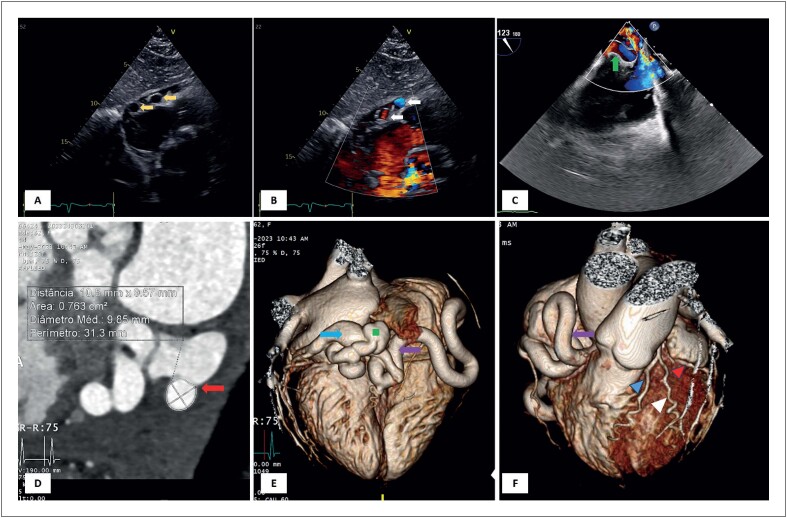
Ectasia da Artéria Coronária Direita e Fístula para o Seio Coronário. Painel A: Ecocardiograma transtorácico (ETT) em vista subcostal, mostrando estruturas pericárdicas redondas (setas amarelas). Painel B: ETT com Doppler, mostrando vascularização das estruturas (setas brancas). Painel C: Ecocardiograma transesofágico mostrando uma artéria coronária direita gigante (ACD) (seta verde) com fluxo turbulento em seu interior. Painel D: Angiografia coronária por tomografia computadorizada (ATC) mostrando uma ACD ectásica com fistulação para o seio coronário (SC) (seta vermelha). Painel E: Vista posterior da ATC mostrando o SC (seta azul), a ACD ectásica (seta roxa) e a localização da fístula (quadrado verde). Painel F: Vista anterior da ATC mostrando a ACD (seta roxa), a artéria descendente anterior (ponta de seta azul), uma artéria marginal (ponta de seta branca) e a artéria circunflexa (ponta de seta vermelha).
